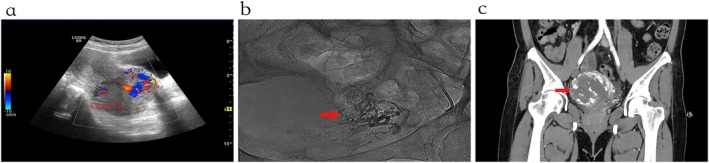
The lesion demonstrated by ultrasonography, abdominal CT and angiograph. a. Heterogeneous mass in the posterior cervical wall with ill‐defined margins and abundant vascular signals. b. Heterogeneous mass in the right posterior cervical wall with multiple enlarged and tortuous vessels on contrast‐enhanced imaging. c. Angiography during uterine artery embolization: Markedly enlarged and tortuous uterine arteries.
